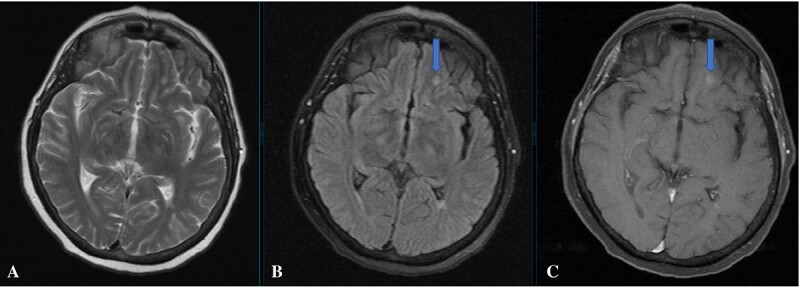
A metastatic nodular lesion was detected in the left frontobasal subcortical white matter which was hyperintense on axial T2-weighted MRI (A) and FLAIR image (B). The lesion also shows contrast enhancement (C). Blue arrows indicate lesions.
